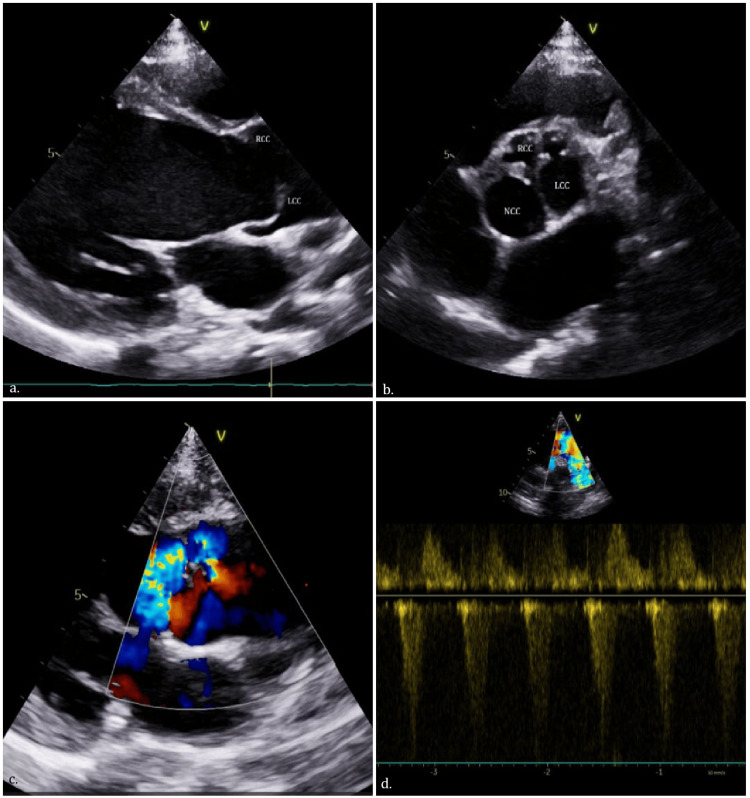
Two-dimensional echocardiography. (a) Parasternal long-axis view showing a hypoplastic RCC and well-formed NCC, with a dilated LV. (b) Parasternal short-axis view demonstrating a trileaflet aortic valve with hypoplastic RCC and well-formed LCC and NCC. (c) Parasternal long-axis view with color Doppler showing a severe posteriorly directed AR jet. (d) Suprasternal view with continuous-wave Doppler of the descending thoracic aorta showing pandiastolic flow reversal, suggestive of severe AR. AR: aortic regurgitation; LV: left ventricle; LCC: left coronary cusp; NCC: non-coronary cusp; RCC: right coronary cusp
